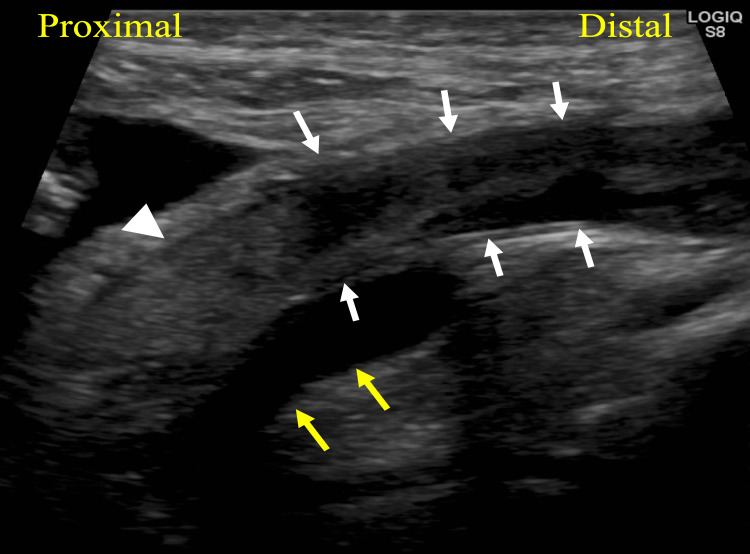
Venous ultrasonography. Lower extremity venous ultrasonography reveals a possible thrombus formation (white arrowhead), as manifested by heavy spontaneous echo contrast (white arrow) distal to the obstruction. White arrows delineate the right external iliac vein and yellow arrows indicate the right external iliac artery.
